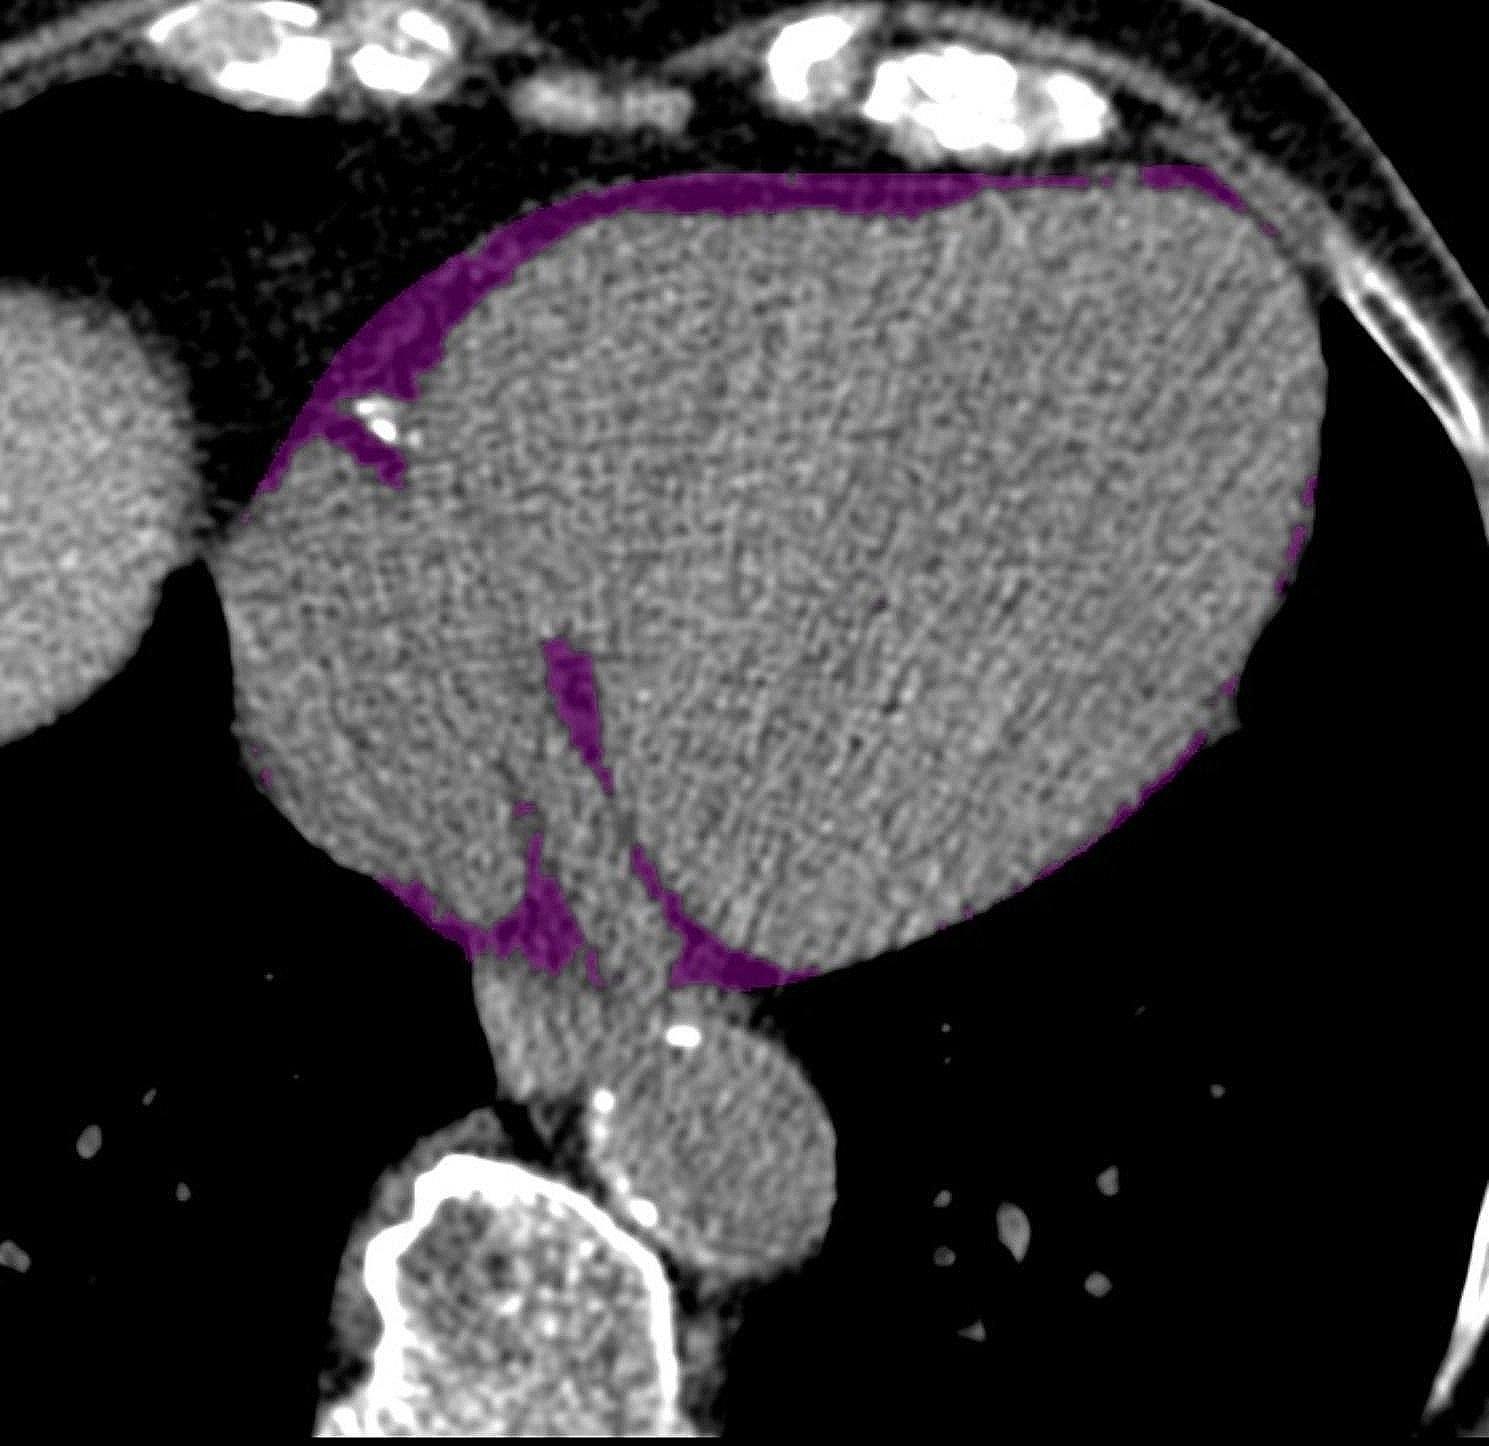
Example of EFT volume measurement. CT-derived slice of the heart in the axial view with tracing of the pericardial border. The areas marked in purple represent EFT. Abbreviations: EFT = epicardial fat tissue; CT = computed tomography
